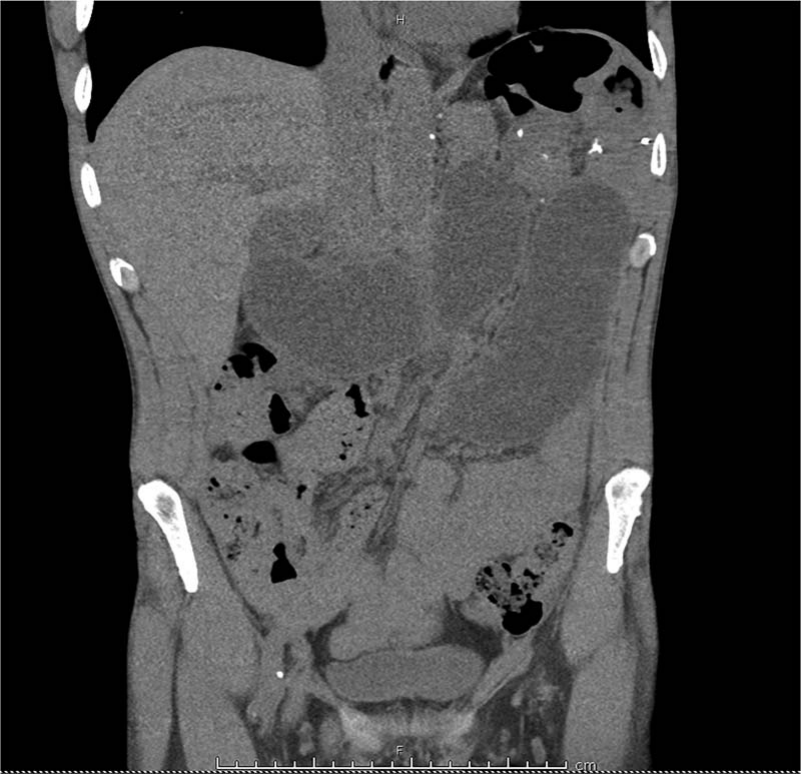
A CT scan of the patient’s abdomen demonstrating obstruction suggestive of internal hernia.
